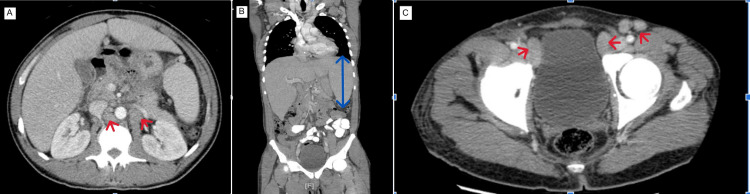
(A) Computed Tomography (CT) chest/abdomen/pelvis with contrast (axial view) showing retroperitoneal lymphadenopathy (red arrows). (B) CT chest/abdomen/pelvis with contrast (coronal view) showing splenomegaly (blue straight line with double arrows). (C) CT chest/abdomen/pelvis with contrast (axial view) showing pelvic and inguinal lymphadenopathy (red arrows).
